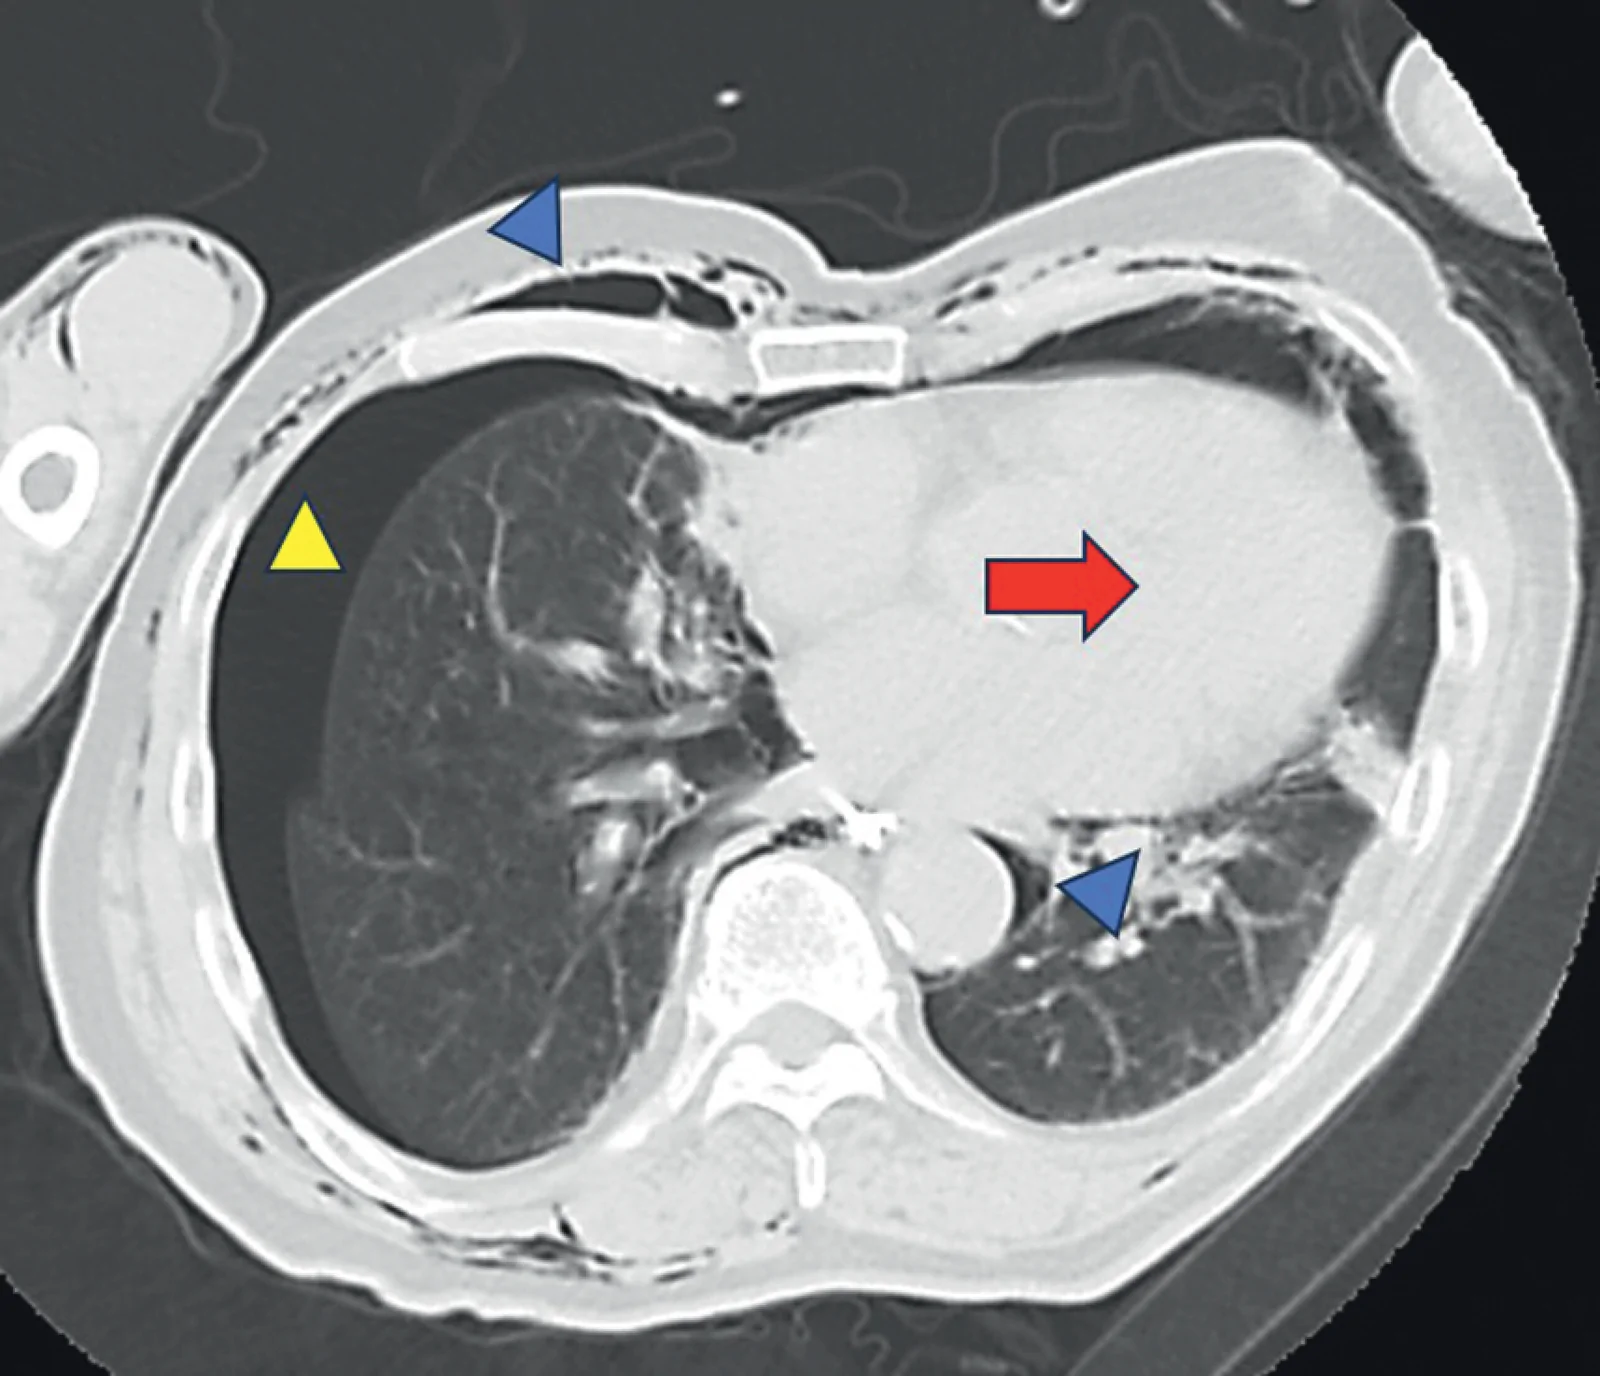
CT performed immediately after endoscopic submucosal
